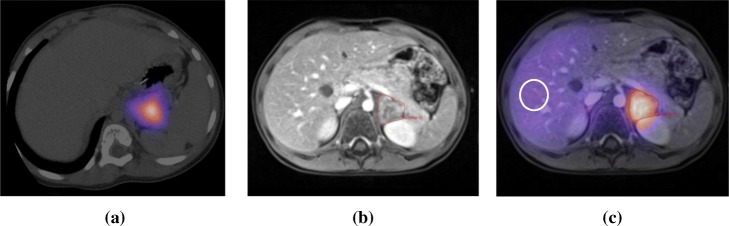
Image data depicting SPECT/CT (a), MRI alone (b) and fused SPECT/MRI (c). The lesion can clearly be seen as the volume with intense uptake adjacent to the left kidney (delineated in b and c)
